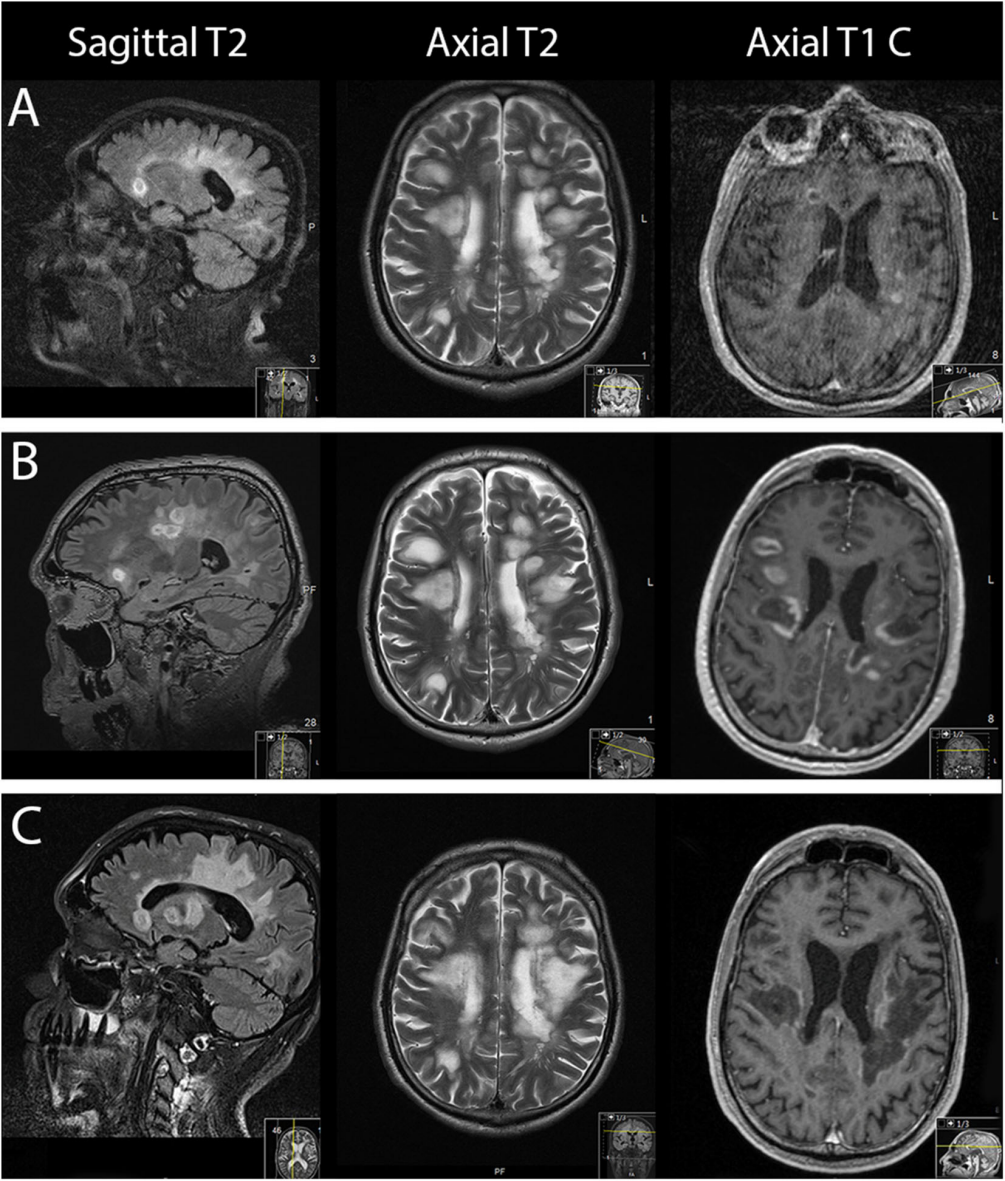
From left to right: sagittal, axial MRI T2-weighted sequences and axial gadolinium contrast T1-weighted sequences. (A) On the day of admission. (B) Three days after admission. (C) Forty-one days after admission.
